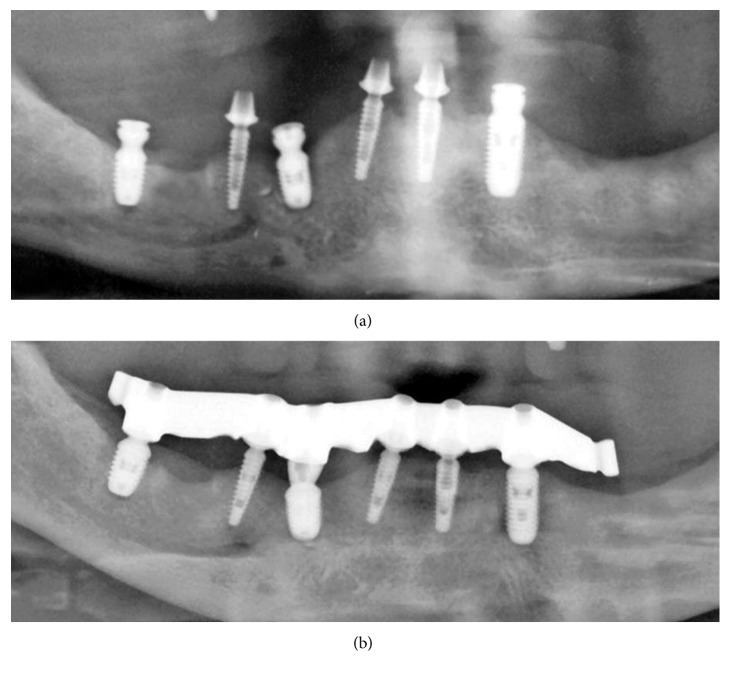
Mandibular OPG sections eight months postoperatively (not modified radiograph taken during current treatment). (a) After placement of three additional implants. (b) After bar mounting.
